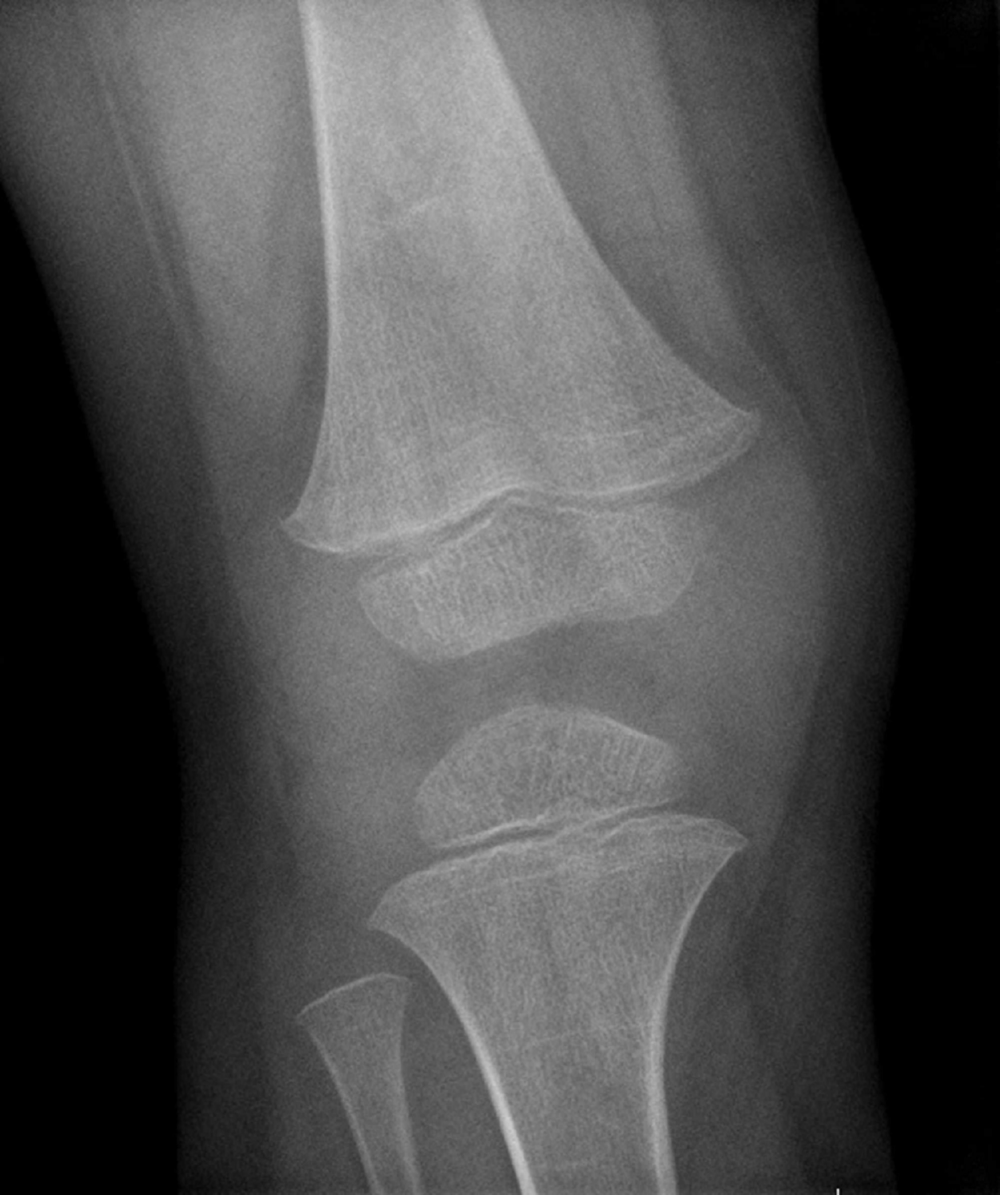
X-ray of the right knee from initial presentation to hospital
